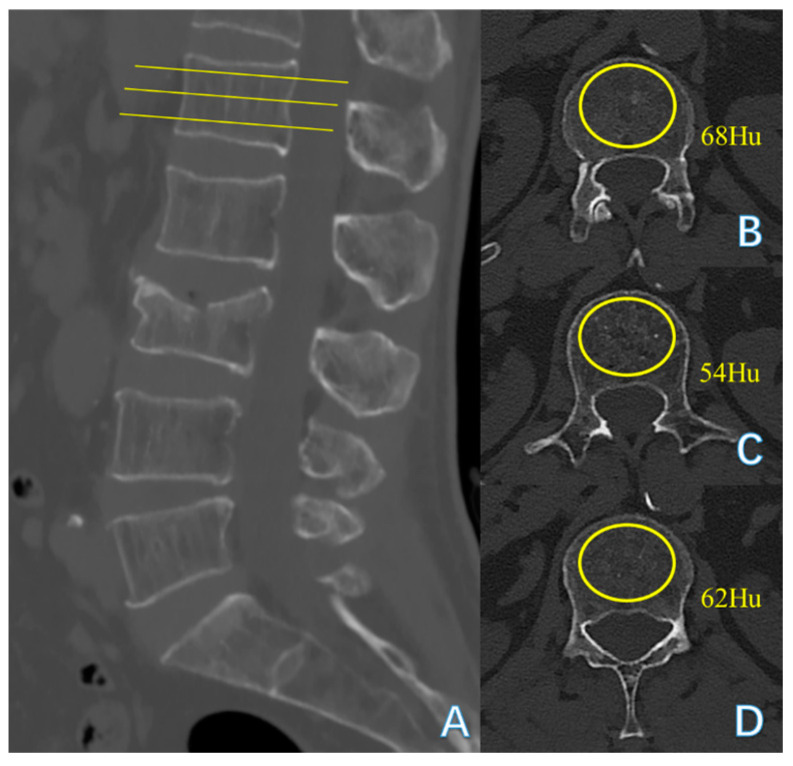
A 70-year-old male patient with compression fracture of L3: (A) midsagittal plane; (B) inferior to the upper endplate; (C) in the middle of the vertebral body; (D) superior to the lower endplate. The Hu on CT was (68 + 54 + 62)/3 = 61.33.
